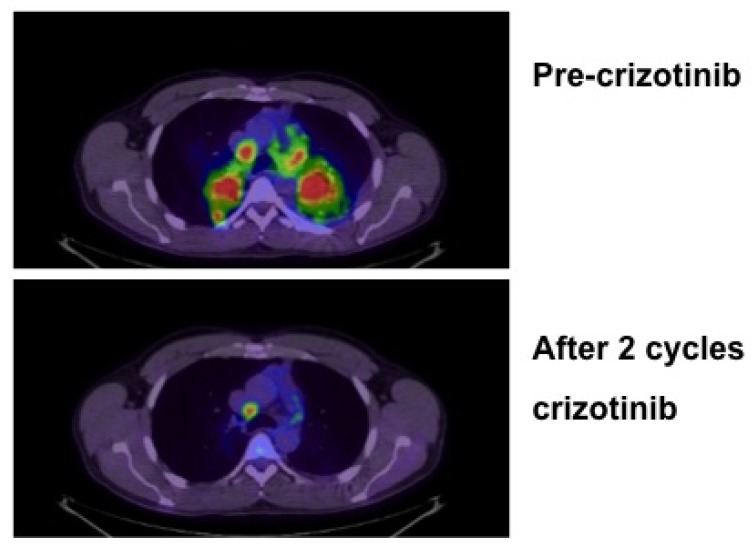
FDG PET/CT scans in a 43 y.o. male never smoker with anaplastic lymphoma kinase (ALK)-positive non-small cell lung cancer (NSCLC) prior to and after two cycles of Crizotinib.
